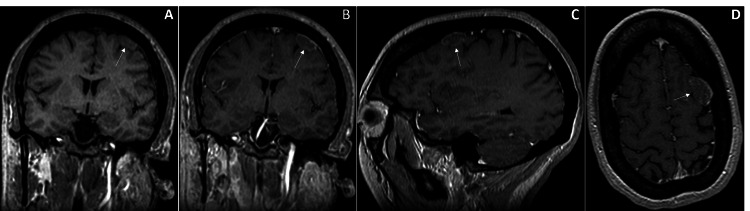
MRI brain further characterizing the left frontal lesion. Pre-contrasted coronal T1 section demonstrating a well-marginated, iso-dense, dural-based, extra-axial round mass lesion along left frontal convexity (A). Post-contrasted coronal (B), sagittal (C), and axial (D) T1 sections re-demonstrating the same left convexity lesion, now with peripheral contrast enhancement.
